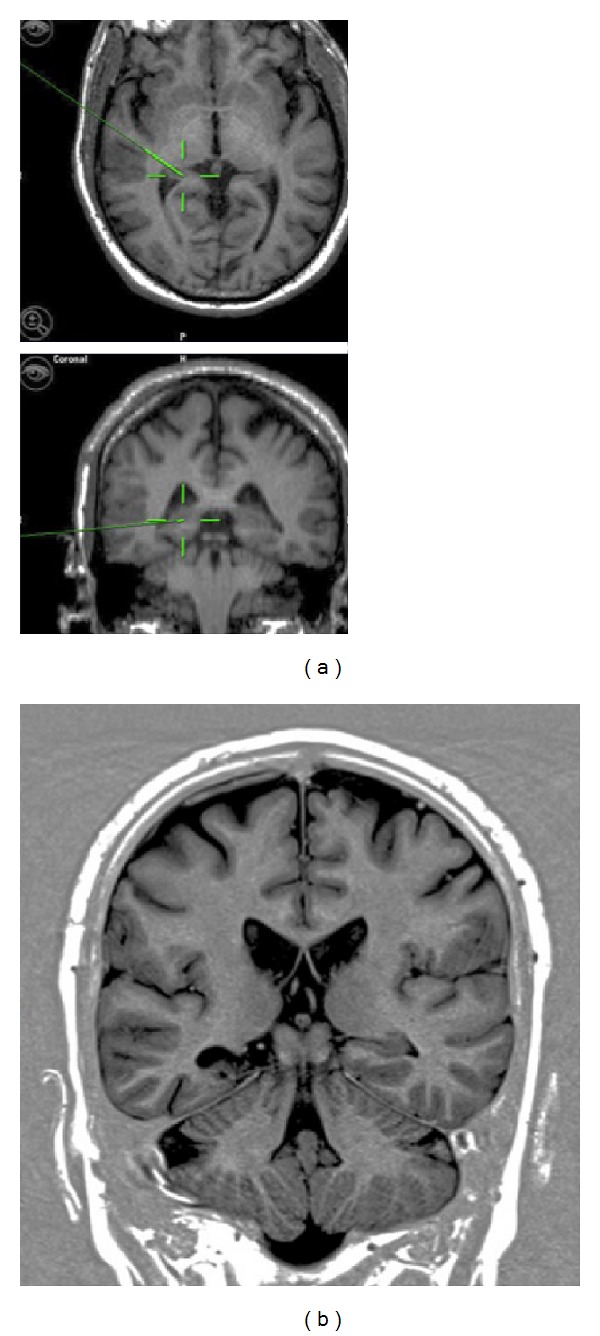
Intraoperative neuronavigation (a) and postoperative MRI brain (b) showing the difference in posterior extent of hippocampus resection. Intraoperative neuronavigation image guidance does overestimate the extent of posterior resection due to intraoperative brain shift.
